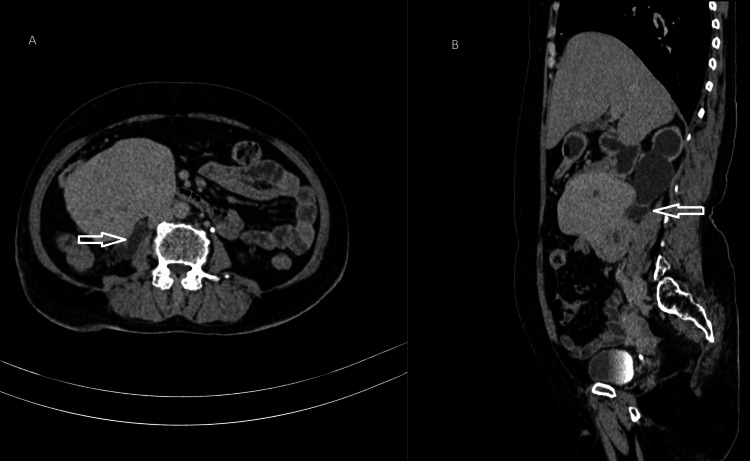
CT urography images of the axial (A) and sagittal (B) sections in the excretory phase showing mass lesions compressing the right proximal ureter (white arrows), causing upstream hydroureteronephrosis. Also, there was no contrast excretion into the right ureter, suggesting a nonfunctioning right kidney, whereas the left ureter showed good contrast excretion CT: computed tomography
